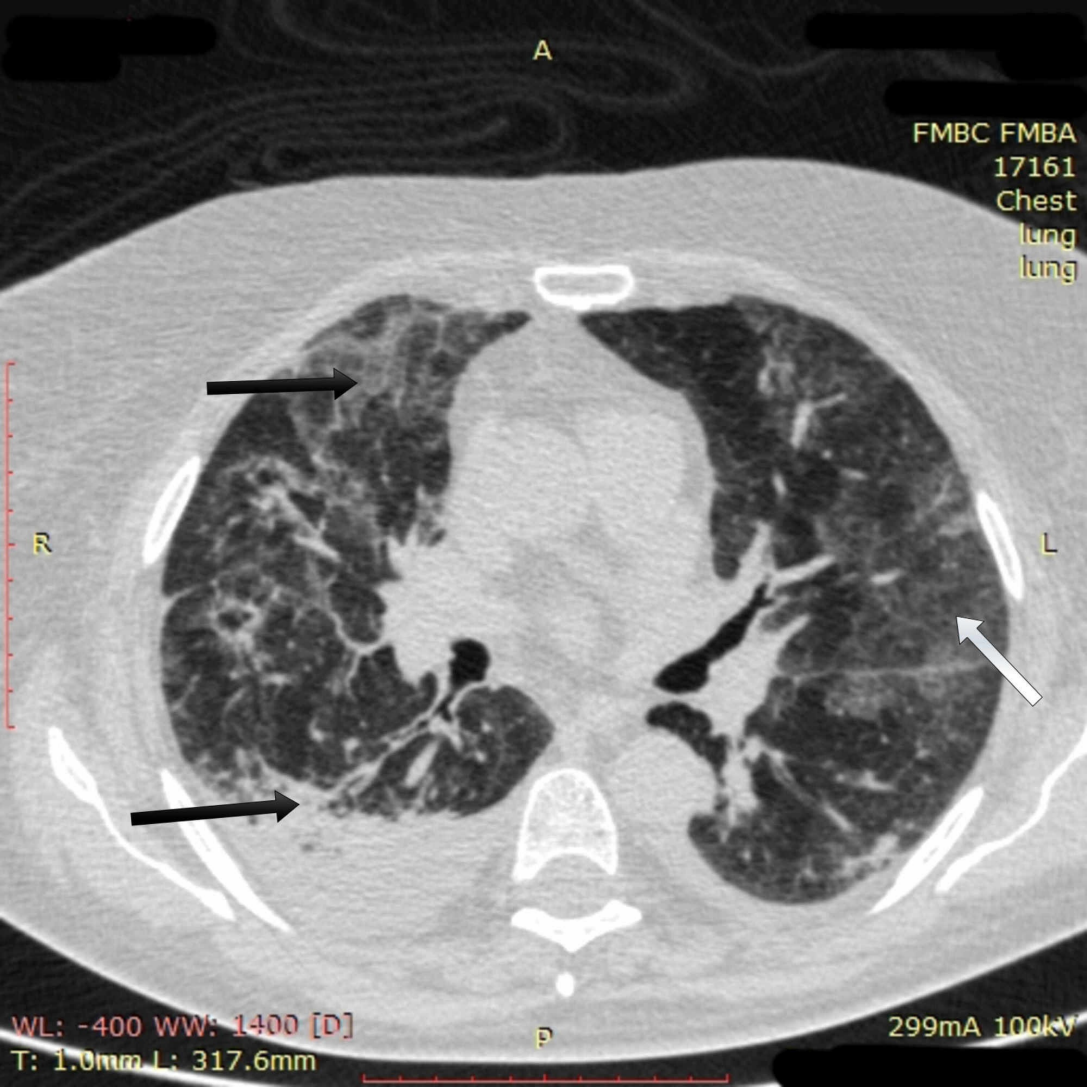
Chest CT imaging of patient 1 on day 17 Chest CT on day 17 showed multiple confluent ground-glass opacities had increased in size (white arrow); bilateral pulmonary infiltrates had lost their density to the degree of ground-glass opacity, and their area had decreased (black arrows) CT: computed tomography
